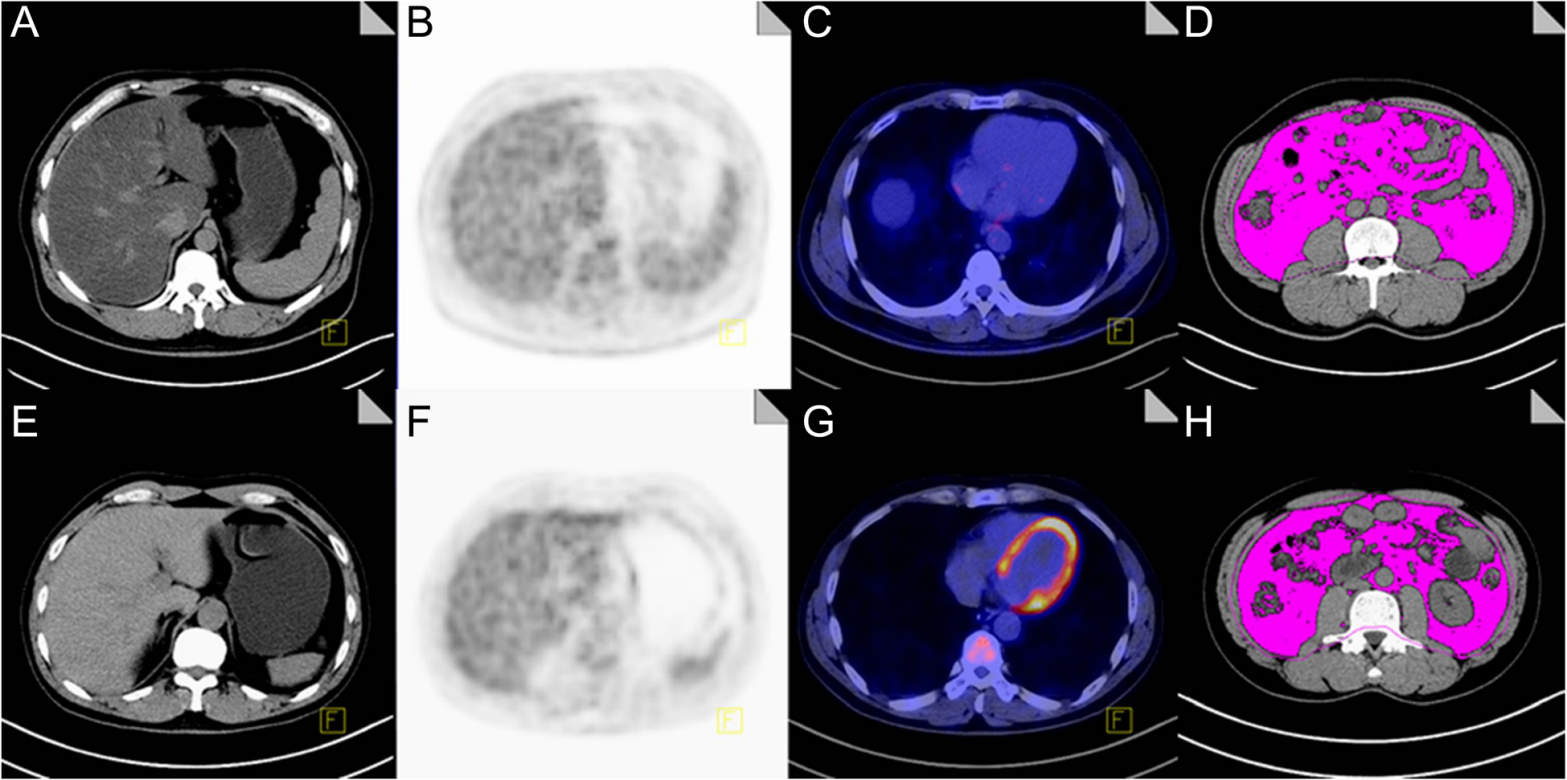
Comparison of PET/CT images between NAFLD patients and non-NAFLD patients. a-d are the images of a 45-year-old male, showing a significantly reduced liver CT density, liver CT value of 15.67 HU, and the ratio of spleen/liver CT intensities of 2.94; b liver SUVmean of 1.55; c no significant FDG uptake in the myocardium; d VAT volume of 2250 cm^3^. e-h are the images of a 50-year-old male, showing e liver CT value of 56.00 HU, and the ratio of spleen/liver CT intensities of 0.74; f liver SUVmean of 1.81; g significant FDG uptake in myocardium; h VAT volume of 1552 cm^3^
